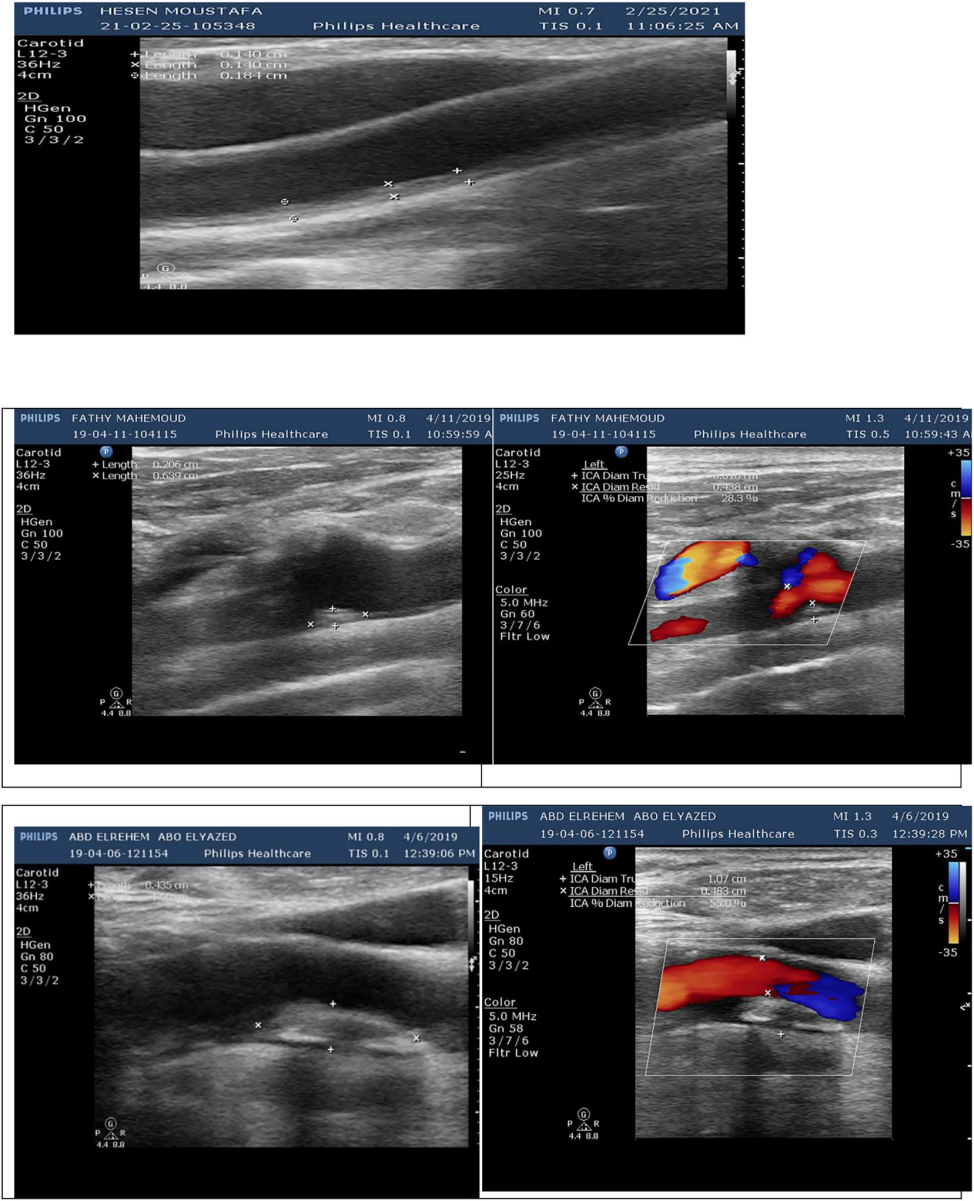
Duplex ultrasonography study in chronic obstructive pulmonary disease (COPD) patients with upper panel showing increased intima–media thickness (IMT) (about 0.15 cm); the second panel showing homogenous hyperechoic focal thickness in the distal common carotid artery (CCA) measuring 0.36 cm × 0.20 cm, making diameter stenosis 28.3%; and the third panel showing homogenous hyperechoic plaque in the internal carotid artery (ICA) measuring 1.00 cm × 0.54 cm, making diameter stenosis 55%
